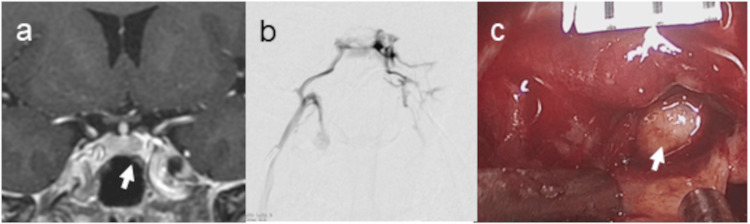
Case 1. A patient in whom venous drainage led to false-lateralization on IPSS, which was not corrected by prolactin adjustment (Table 1). A 33-year-old woman. An SPGR MR image shows equivocal lesions on the left side (arrow) (a). Venography from the left IPS demonstrated dominant venous drainage from the left to the right side (b). Intraoperative image demonstrates tumor located in the left lateral wing (arrow) (c). IPSS: inferior petrosal sinus sampling, SPGR: spoiled gradient echo sequence
